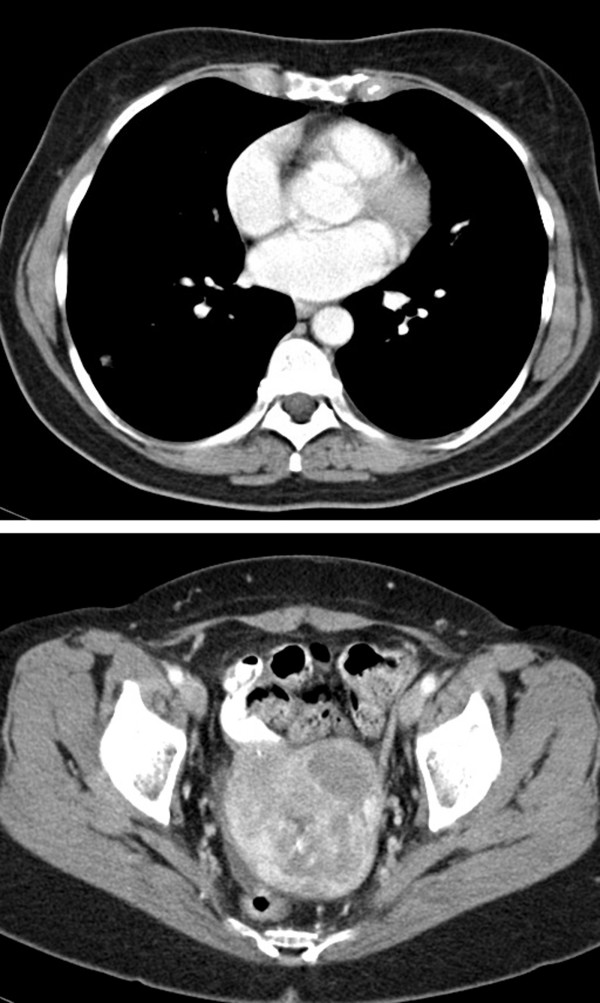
CT scan at start of chemotherapy cycle #8.
